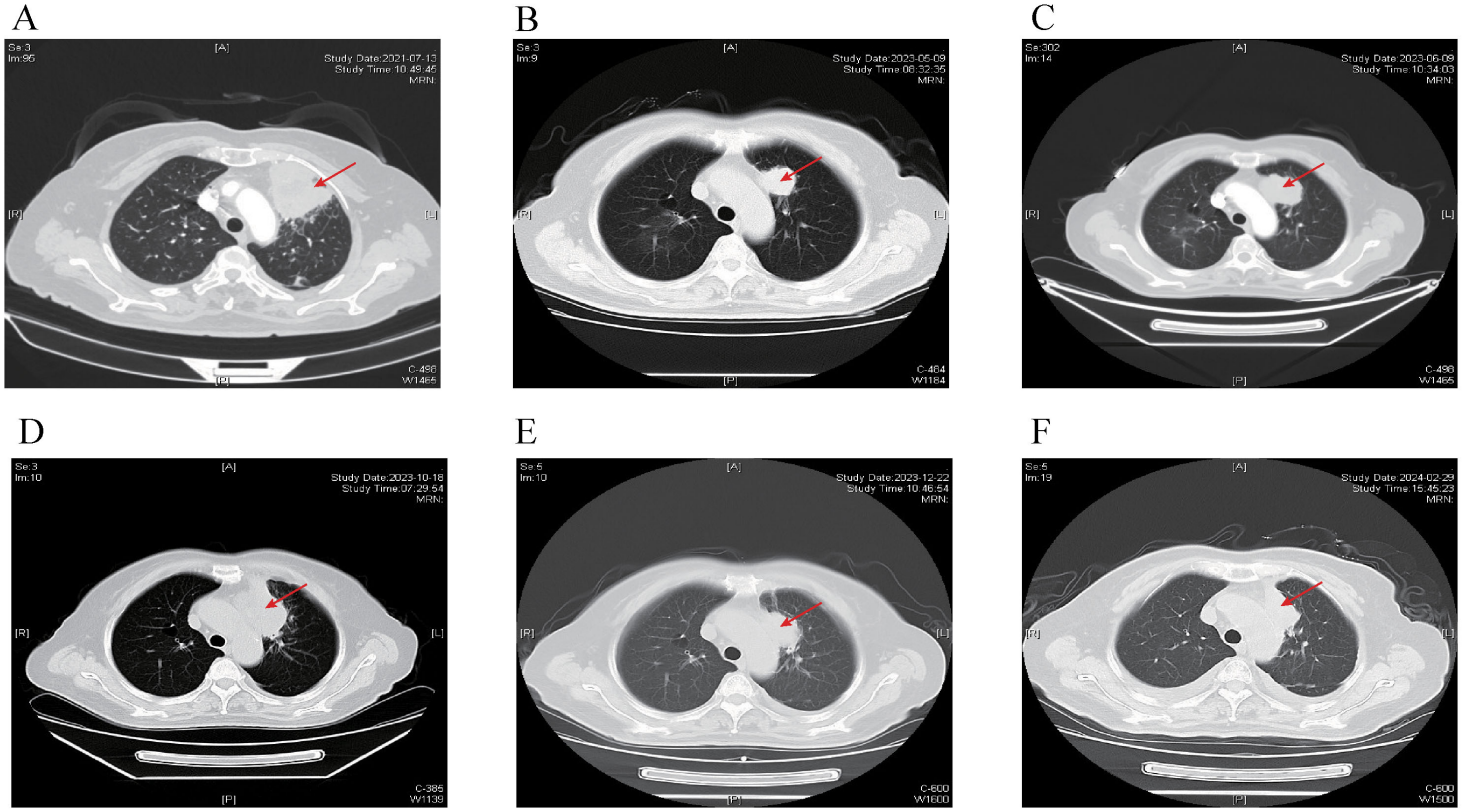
Chest CT scans at different time points. The red arrow indicates primary lesion in the left upper lobe of the lung. (A) Chest CT scan of baseline. (B) Chest CT scan of best response PR after first-line treatment with Osimertinib. (C) Chest CT scan showing progression after 24 months of Osimertinib. (D) Chest CT scan showing progression after 4 months of Anlotinib and Aumolertinib. (E) Chest CT scan showing reduction in the LUL lesion after 2 cycles of EP chemotherapy plus Adebrelimab. (F) Chest CT scan showing regression in the LUL lesion after fourth-line treatment with Osimertinib in addition to the existing chemotherapy and immunotherapy regimen. CT, computed tomography; PR, partial response; LUL, left upper lobe; EP, etoposide plus cisplatin.
